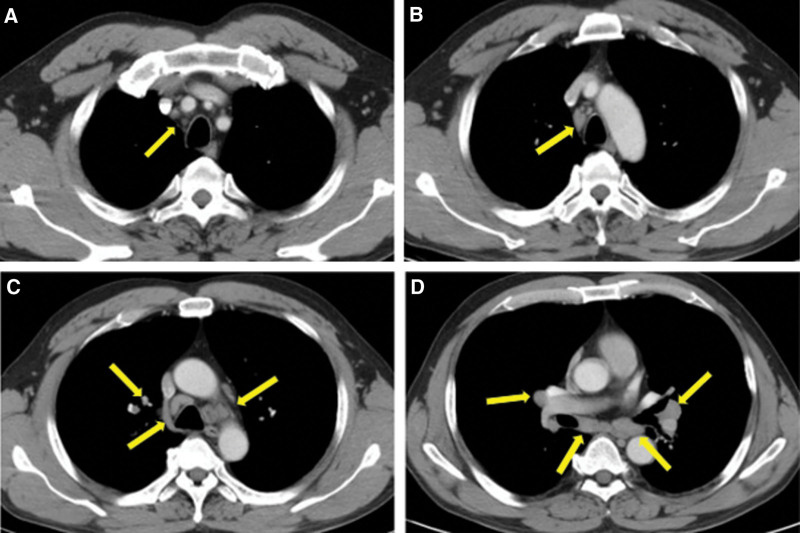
Computed tomography scan after two cycles of sintilimab treatment of the case report patient showing multiple enlargement of mediastinal and bilateral hilar lymph nodes (yellow arrows).
